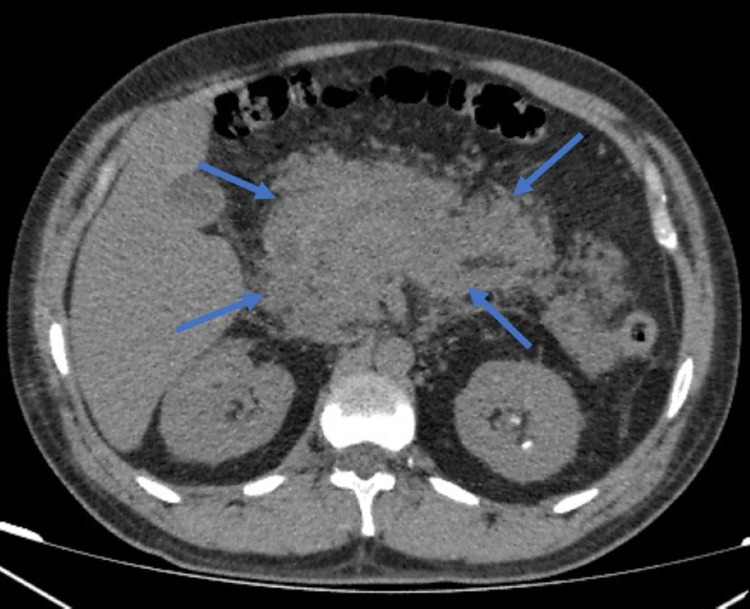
Abdomen CT demonstrating diffuse enlargement of pancreas with ill-defined borders (arrows)
